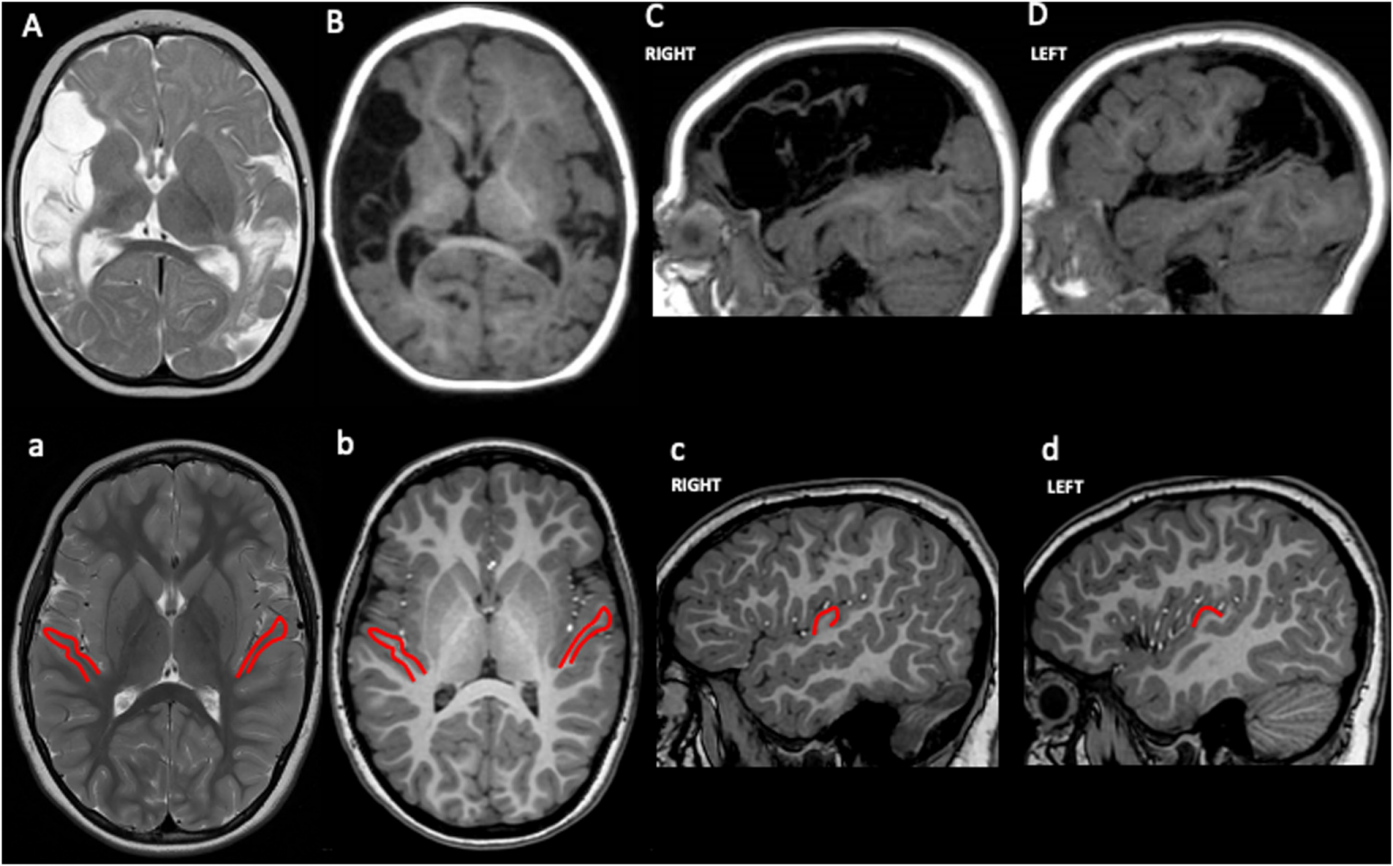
Magnetic resonance imaging of the patient with presumed perinatal bilateral stroke (A–D) and of an age-matched healthy subject (a–d). Axial T2-weighted (A) and axial (B) and sagittal (C, D) T1-weighted images shows malacic and cystic areas with an asymmetrical distribution (right > left) involving cortical territories of middle cerebral artery. Axial T2-weighted (a) and axial (b) and sagittal (c, d) T1-weighted images shows normal anatomy; in-plane projections of Heshl gyrus in the superior temporal gyrus are delineated in red.
